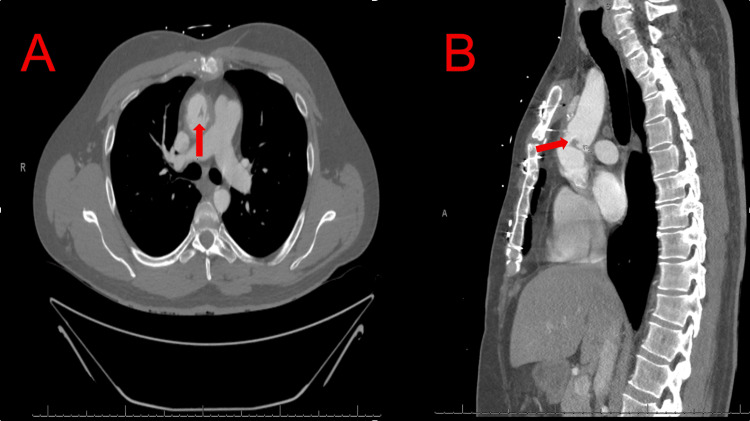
Computed Tomography Angiography Demonstrating an Intraluminal Lesion at the Ascending Aortic Graft Computed tomography angiography of the chest with intravenous contrast demonstrating an intraluminal lesion at the ascending aortic graft. Panel A (axial view) shows a focal irregular hypodense lesion projecting into the lumen of the ascending aortic graft (arrow). Panel B (sagittal reconstruction) localizes the lesion to the distal anastomotic site at the junction of the graft and native aorta (arrow). No periaortic fluid collection, pseudoaneurysm, or aortic dissection is identified.
